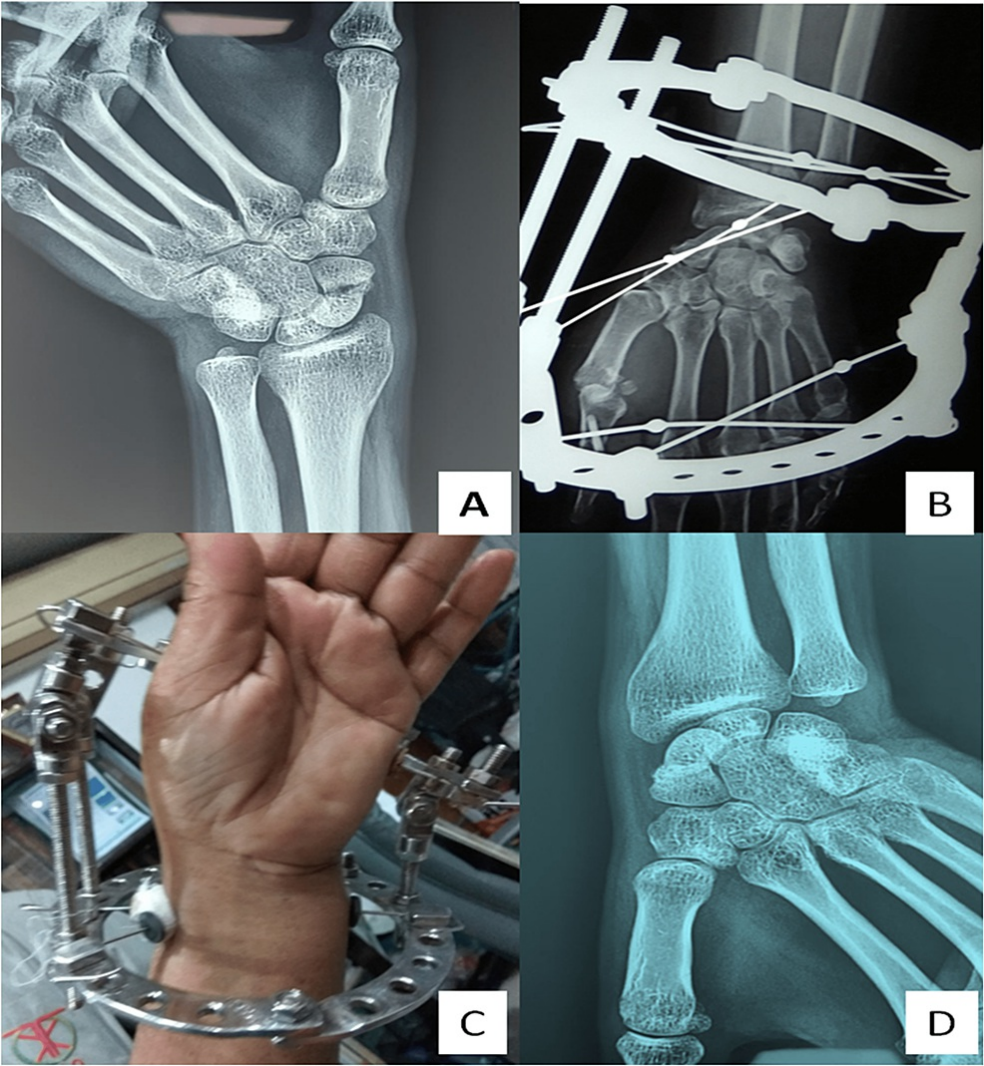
SNU in patient number 4, a preoperative radiograph (A) and a postoperative X-ray (B) and healed SNU radiograph in patient number 4 (D). SNU, scaphoid nonunion
